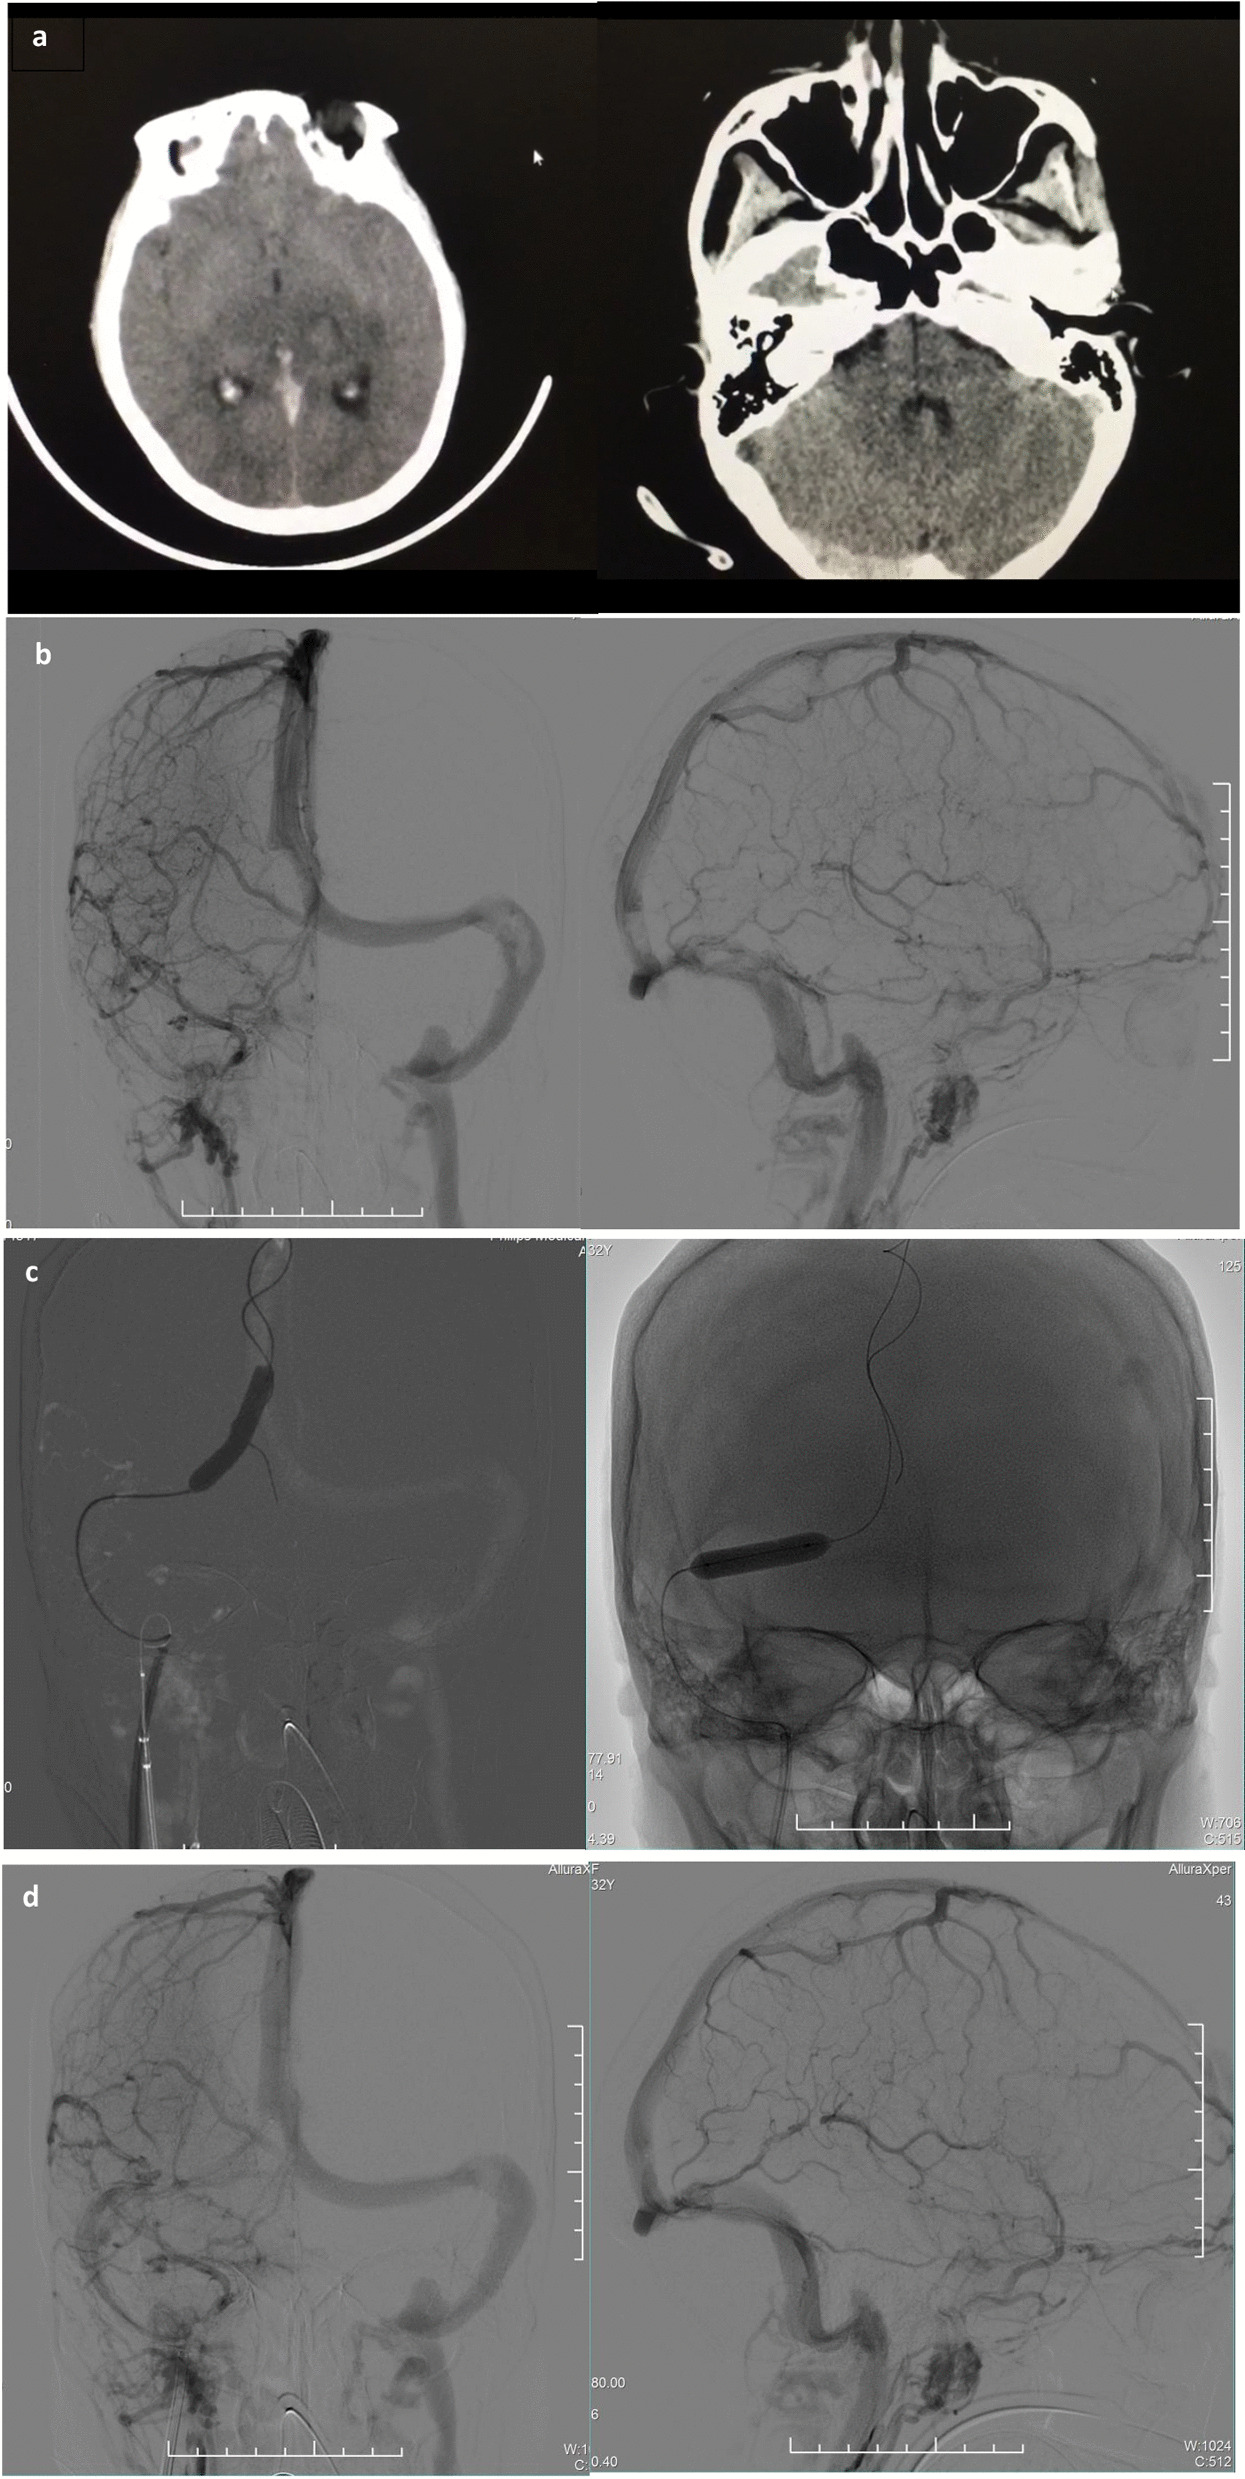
a Noncontrast brain CT showing increased density of straight sinus and right transverse sinus. b The anterior and lateral positions of the right internal jugular vein stage: nonvisualization of right transverse, sigmoid sinus, and straight sinus, and identification of large thrombus among the junction of the superior sagittal sinus, the left transverse sinus, and the sigmoid sinus, with severe stenosis of the corresponding vein lumens. c Balloon-dilated sinus confluence and transverse sinus. d Final angiography showing improvement of the right transverse sinus and the sigmoid sinus compared with the front, and nonvisualization of the straight sinus
